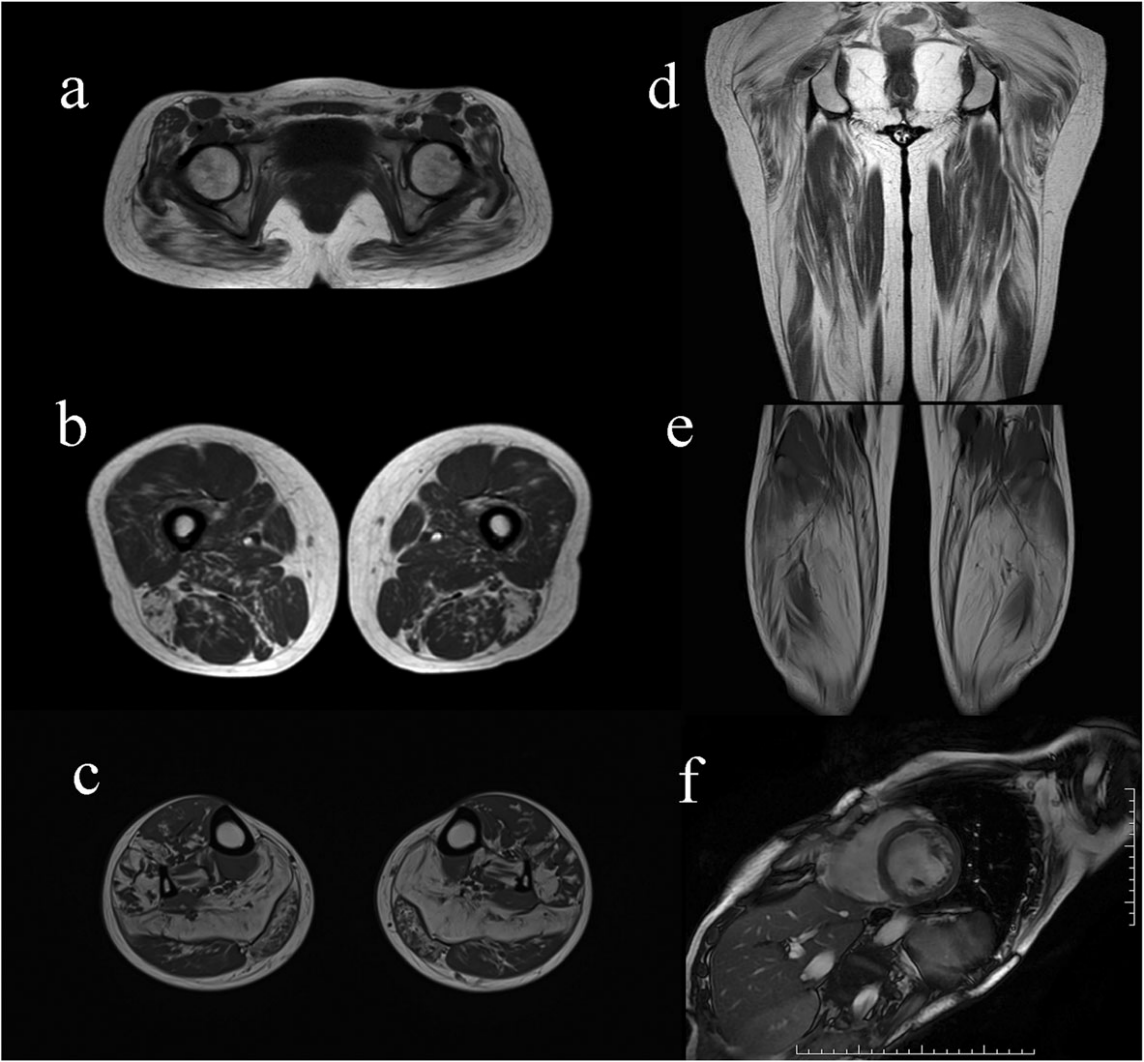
Lower leg muscle and cardiac MRI characteristics in NLSDM patients. a MRI revealed diffuse fatty infiltration, predominantly involving the gluteus maximus at the pelvic level. b, d The long head of the biceps femoris, semimembranosus and adductor magnus were moderately-to-severely affected. The rectus femoris, gracilis and sartorius were relatively preserved. c, e The soleus and medial head of the gastrocnemius were severely affected. The anterior and posterior tibialis were relatively preserved. (f) Cardiac MRI showed dilated cardiomyopathy
